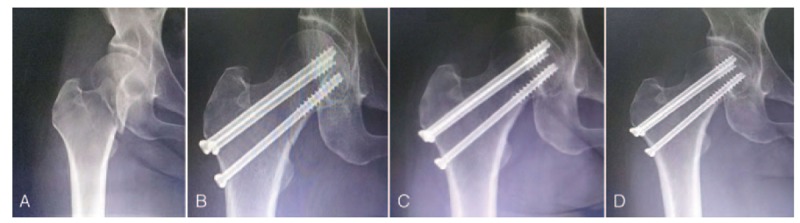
Fluoroscopic anteroposterior view of the displaced femoral neck fracture fixed with parallel cannulated screws, taken at different times. (A) Preoperative X-ray, (B) postoperative X-ray on the day of surgery, (C) postoperative X-ray at 6 months, (D) postoperative X-ray at 15 months.
